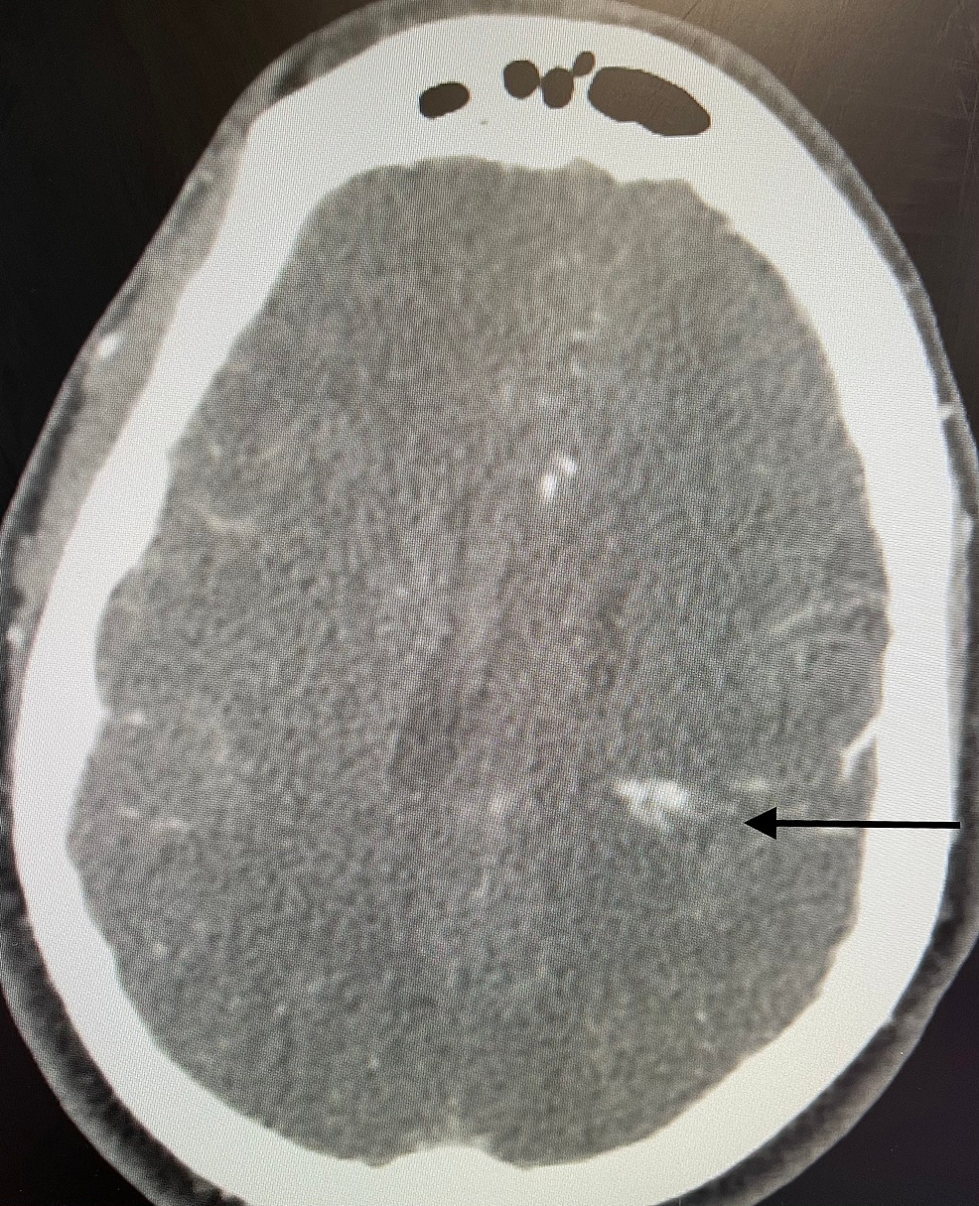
CTA demonstrating left parietal arteriovenous malformation (arrow) CTA: computed tomography angiography
